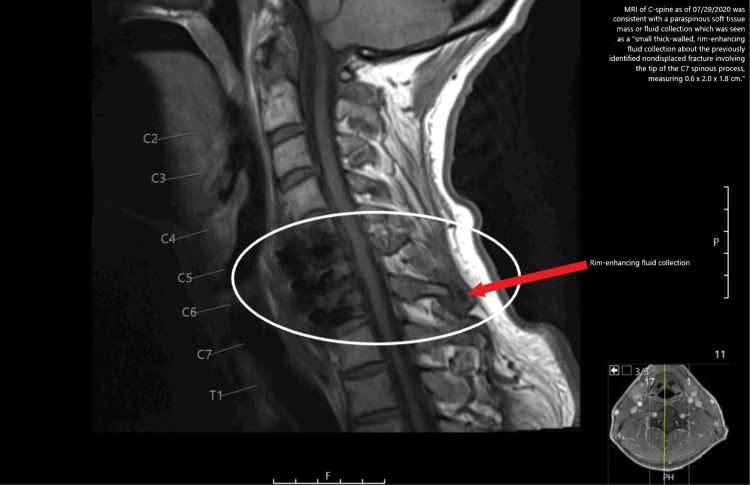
MRI scan of C-spine as of September 29, 2020 indicates a small thick-walled, rim-enhancing fluid collection about the previously identified nondisplaced fracture involving the tip of the C7 spinous process and measuring 0.6 × 2.0 × 1.8 cm. MRI: magnetic resonance imaging
